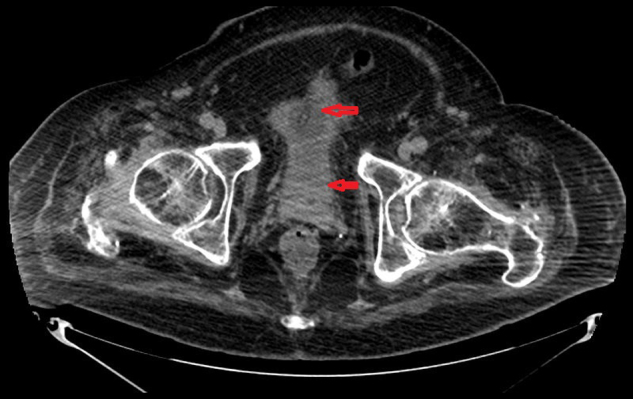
Computed tomography of pelvis performed in October 2014 (about 7 years after spinal cord injury) showed urinary bladder (bottom arrow) completely collapsed around the balloon (top arrow) of the suprapubic catheter
